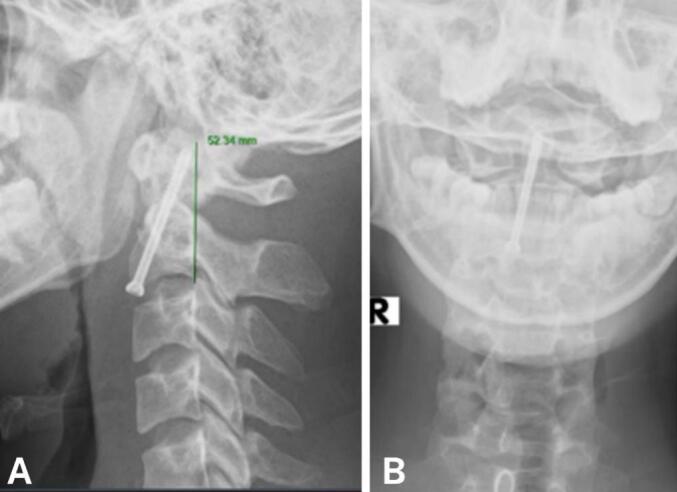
Postoperative X-ray shows the screw placed in the correct position, penetrating the odontoid process to fix the fracture firmly.
